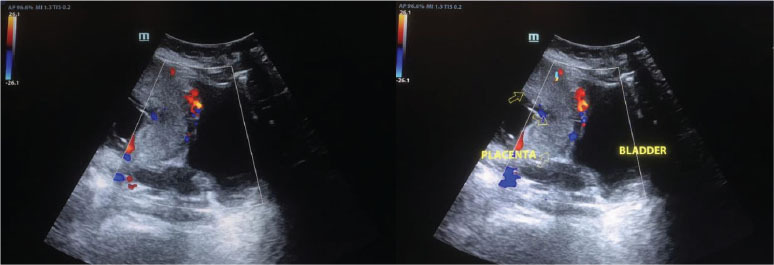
Colour Doppler ultrasound images showing protrusion of placental tissues beyond the outer confines of the uterine myometrium, with thinning of the uterine serosa-bladder wall complex and increased vascularity between the serosa and the bladder.
